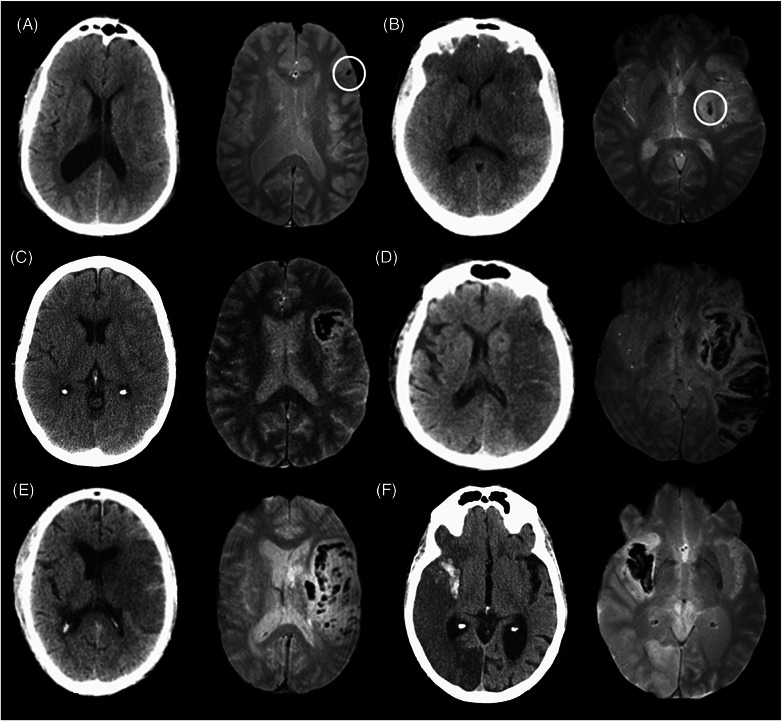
Examples of discordant Heidelberg Bleeding Classification of hemorrhagic transformation and intracranial hemorrhage between CT and MRI. A, HBC 0 on CT, 1a on MRI: 57‐year‐old male, admission NIHSS score 23, M2 MCA (mTICI 3), CT 10 hours before MRI. B, HBC 0 on CT, 1a on MRI: 36‐year‐old female, admissionNIHSS score 28, IV alteplase, M2 MCA (mTICI 2a), CT 9 hours after MRI. C, HBC 0 on CT, 1b on MRI: 69‐year‐old female, admission NIHSS score 17, IV alteplase, M2 MCA (mTICI 2b), CT <1 hour before MRI. D, HBC 1a on CT, 1b on MRI: 75‐year‐old male, admission NIHSS score 26, IV alteplase, M1 MCA (mTICI 3), CT 10 hours before MRI. E, HBC 3c (SAH) on CT, 1b on MRI: 61‐year‐old male, admission NIHSS score 17, eICA (mTICI 2b), CT 8 hours before MRI. F, HBC 1c on CT, 1c and 3C (SAH) on MRI: 62‐year‐old male, admission NIHSS score 25, M1 MCA (mTICI 2b), CT 1 hour after MRI. CT indicates computed tomography; eICA, extracranial internal carotid artery; HBC, Heidelberg Bleeding Classification; IV, intravenous; MCA, middle cerebral artery; MRI, magnetic resonance imaging; mTICI, modified Thrombolysis in Cerebral Infarction; NIHSS, National Institutes of Health Stroke Scale; and SAH, subarachnoid hemorrhage.
